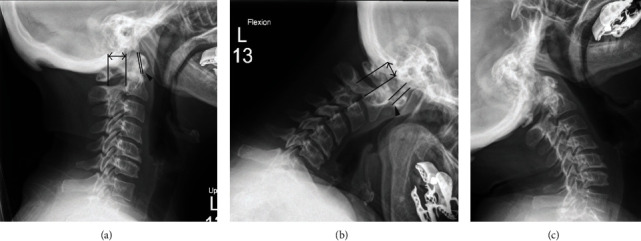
(a) Lateral radiographs of a patient with AAI. In the neutral view, the AAI (arrowhead) is 1 mm. (b) In flexion, the AAI increases to 7 mm. (c) In extension, the AAI reduces to their neutral measures [1].
